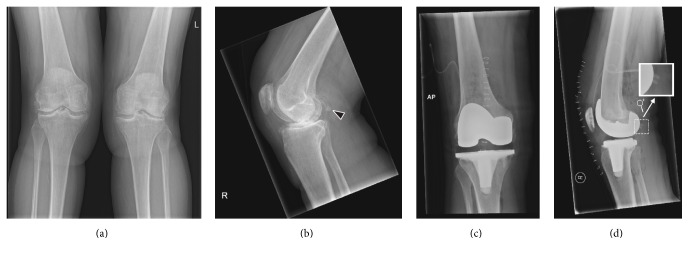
Preoperative (a, b) and immediate postoperative (c, d) knee radiographs in a 68-year-old woman who underwent total knee arthroplasty of the right knee because of symptomatic knee osteoarthritis with valgus malalignment. Preoperative knee radiographs (anterior-posterior view of both knees (a) and lateral view of the right knee (b)) show osteophytes, sclerotic changes, and some degree of generalized joint space narrowing on both sides, in keeping with femorotibial osteoarthritis. There is approximately 10° valgus malalignment of the right knee and approximately 14° valgus malalignment of the left knee. Note the normal appearing fabella ((b) arrowhead). Immediate postoperative radiographs of the right knee on the same day of surgery (anterior-posterior view (c) and lateral view (d)) show not only a good position of the total knee arthroplasty with a well-aligned knee joint but also a horizontal fracture through the fabella with only minimal dislocation ((d) including magnified view of the fabella).
